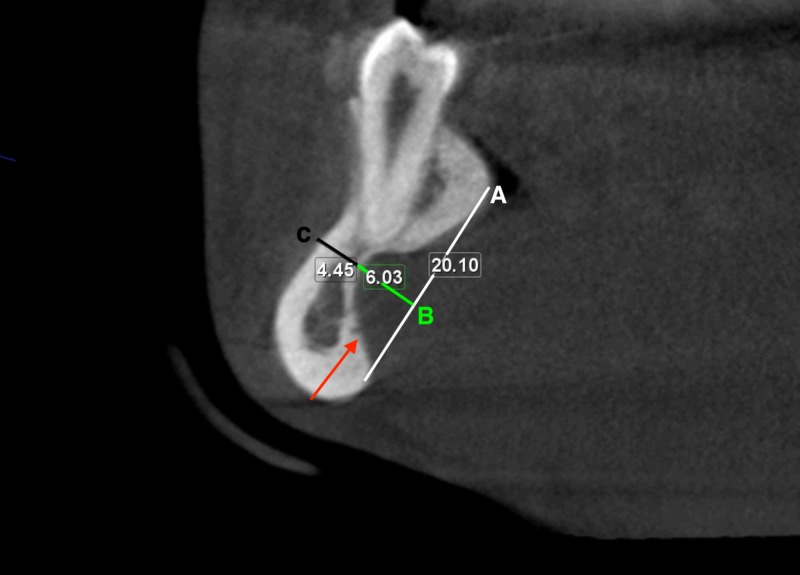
Cross-sectional cone beam computed tomography of the right lingual depression Lingual depression (red arrow), line tangent to the heights of contours of the lingual surfaces of the mandible (A), the deepest point of the depression (B), the buccolingual thickness of the remaining mandible (C).
